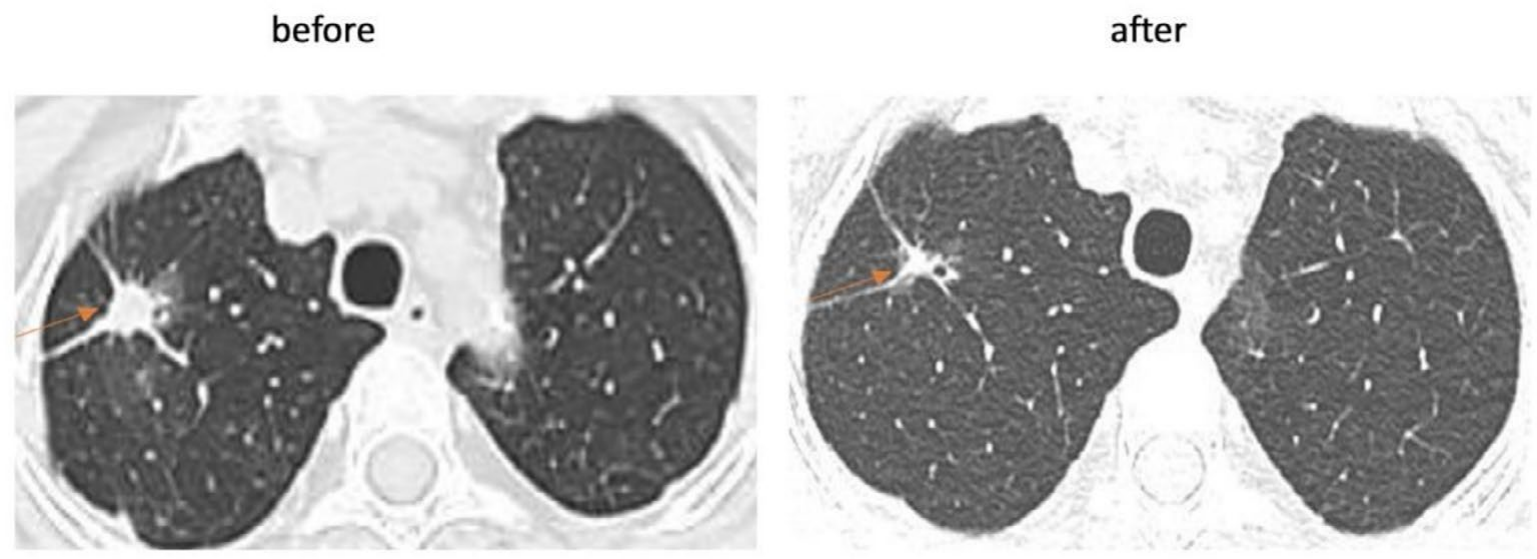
CT scan after 3 months of afatinib therapy. CT scan shows significant size reduction of the right upper lobe tumor after 3 months.
